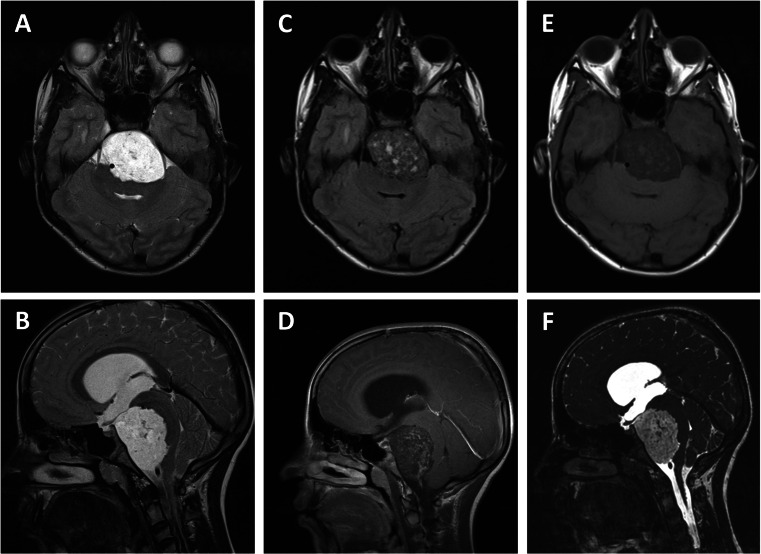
Preoperative MR images. a, b Axial and parasagittal T2-weighted MRI scans showing an inhomogeneous hyperintense prepontine lesion with a mass effect on the brainstem. c, d Axial and parasagittal T1-weighted MRI scan after administration of gadolinium contrast show inhomogeneous enhancement. e Axial T1-weighted MRI scan shows an inhomogeneous hypointense prepontine lesion. f Parasagittal constructive interference steady state (CISS) MRI scan shows an inhomogeneous hyperintense prepontine lesion
